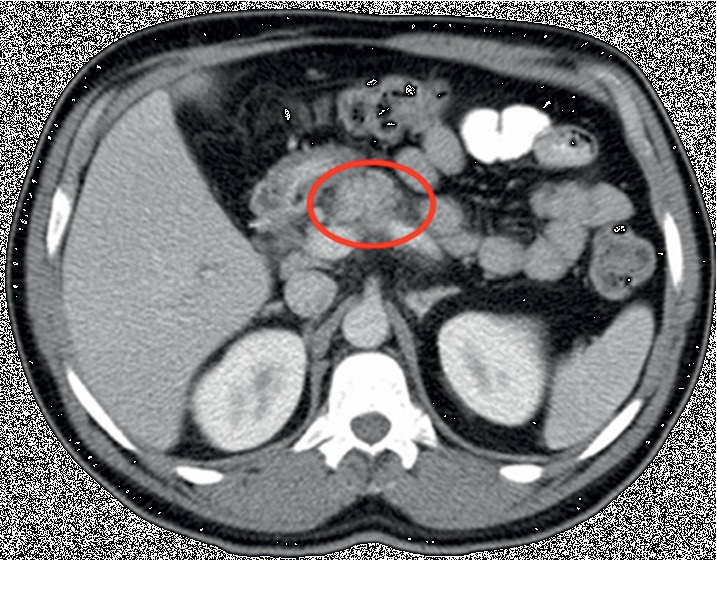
CT scan after subsequent episode of acute pancreatitis.
